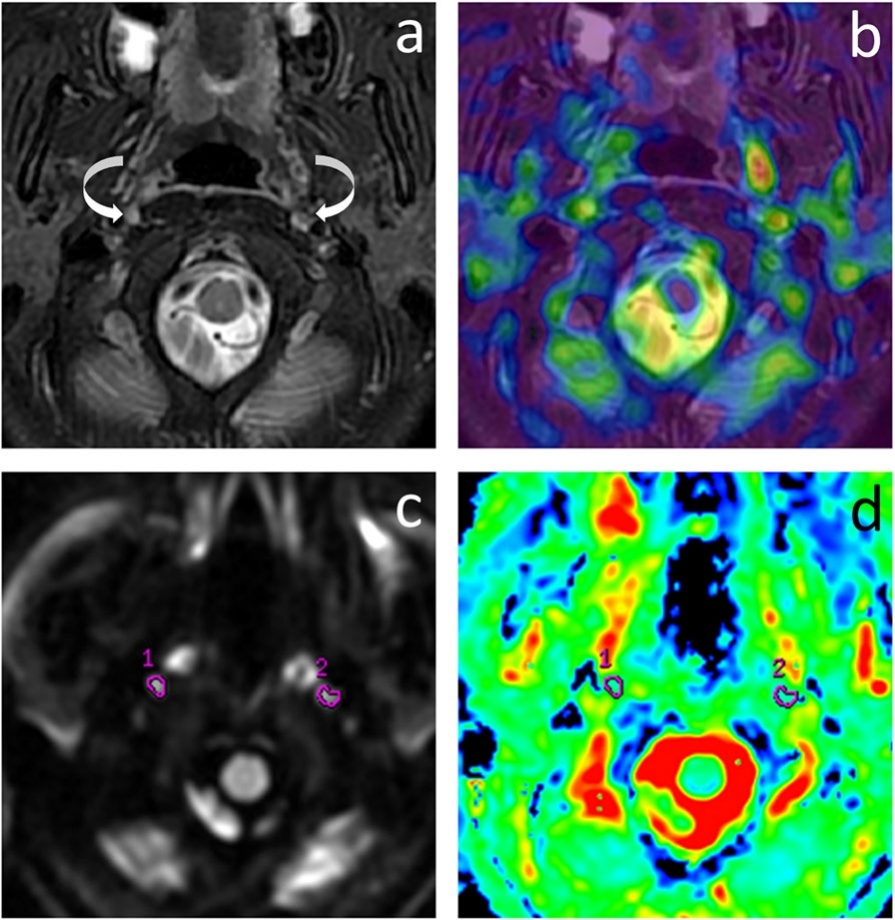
F28, healthy control with bilateral non-metastatic RLNs. Axial T2WI (a) shows bilateral RLNs (curved arrows) with heterogeneous slightly high signal intensity and size as long axis × short axis of 5 × 4 mm (right) and 7 × 5 mm (left), respectively. Both lymph nodes showed slightly higher perfusion compared with the surrounding tissue on the fused image by ASL and T2WI (b), with a BF value of 42.5 mL/100 g/min and 32.14 mL/100 g/min. ROI of the lymph nodes was delineated on the multi-b-value DWI with b value = 800 s/mm^2^
